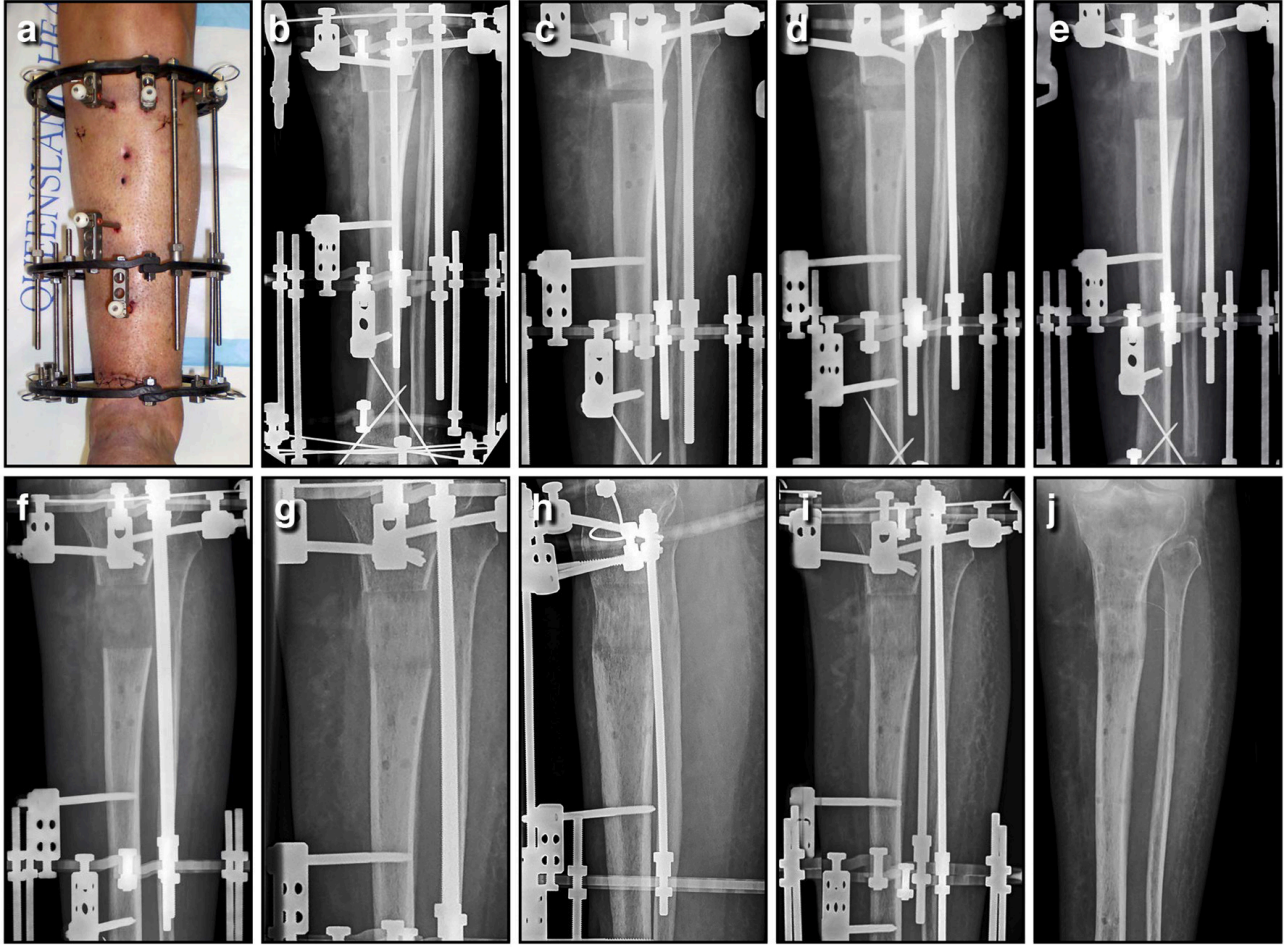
Bone lengthening can be achieved through distraction osteogenesis using an Ilizarov external fixator. In this instance, an open distal tibial fracture resulted in a 3.7 cm segmental defect; the limb was acutely shortened, the wound closed primarily, and length gradually restored through a proximal corticotomy. This panel features a series of AP tibial radiographs demonstrating the 6 month process, and the final result a full year later; (A) Clinical image of the fractured limb stabilized with a circular frame after acute shortening of 3.7 cm; (B) AP radiograph immediately post-operative, with the proximal corticotomy minimally displaced; (C) AP radiograph of proximal tibia lengthening after 2 weeks of distraction; (D) AP radiograph of a proximal tibia lengthening at 4 weeks, with regenerate bone visible in the distraction gap; (E) AP radiograph of a proximal tibia lengthening at 6 weeks, as the gap slowly increases in size; (F) AP radiograph of a proximal tibia lengthening at 8 weeks, after length has been restored; (G) AP radiograph of a proximal tibia lengthening at 12 weeks, as the new bone gradually matures; (H) AP radiograph of a proximal tibia lengthening at 18 weeks, as the bone continues to consolidate; (I) AP radiograph of a proximal tibia lengthening at 26 weeks, shortly before the regenerate bone was solid enough to allow removal of the frame; (J) AP radiograph of the lengthened proximal tibia at 52 weeks, as the bone continues to strengthen and remodel. This regenerate bone will eventually become indistinguishable from surrounding normal bone.
